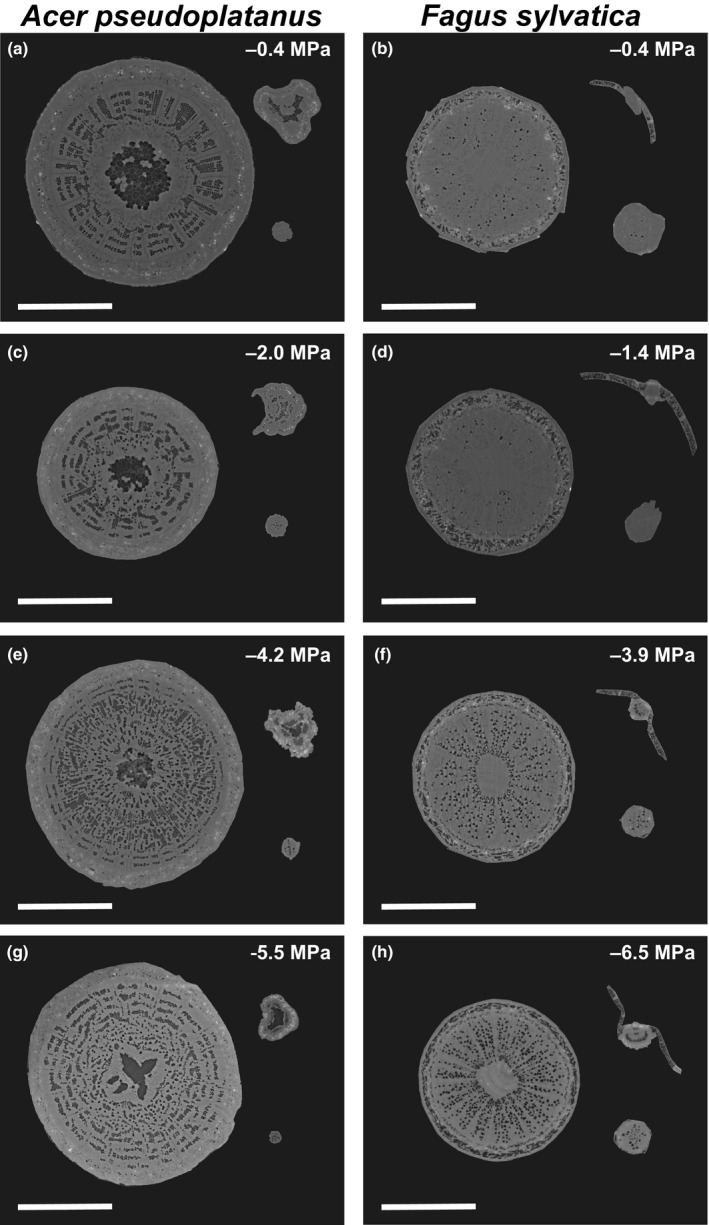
In vivo* visualization by micro‐CT of xylem embolism in stems (left in each panel), leaves (petiole/main vein; upper right in each panel) and roots (lower right in each panel) in intact *Acer pseudoplatanus* (a, c, e, g) and *Fagus sylvatica* (b, d, f, h) seedlings. Reconstructed cross‐sections show embolised (dark grey) and water‐filled (light grey) xylem conduits at different xylem water potential (MPa). Bars, 1250 μm.
